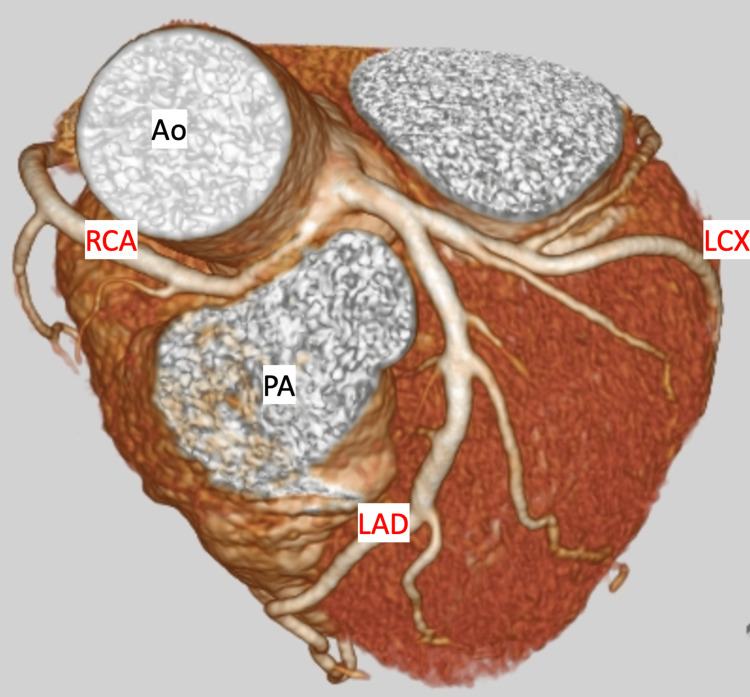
Preoperative coronary computed tomography The right coronary artery originated from the left sinus of Valsalva and it traveled between the ascending aorta and pulmonary artery. LAD, left anterior descending artery; LCX, left circumflex artery; RCA, right coronary artery; Ao, aorta; PA, pulmonary artery.
